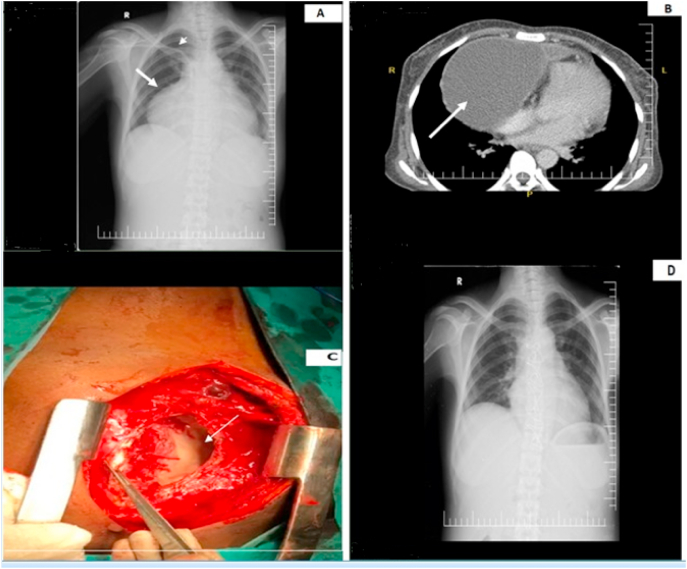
(A) Chest x-ray on admission showing protrusion of the right heart border and enlarged cardiac silhouette. There is also right subclavian hemodialysis catheter. (B) Contrast-enhanced computed tomography scan (CT-scan) of the chest, demonstrating a localized massive pericardial abscess on the right anterior side of the heart. (C) Intraoperative image of localized pericardial abscess (arrow) after a median sternotomy. (D) Chest x-ray on five weeks after operation showing normal cardiac size and recovery.
